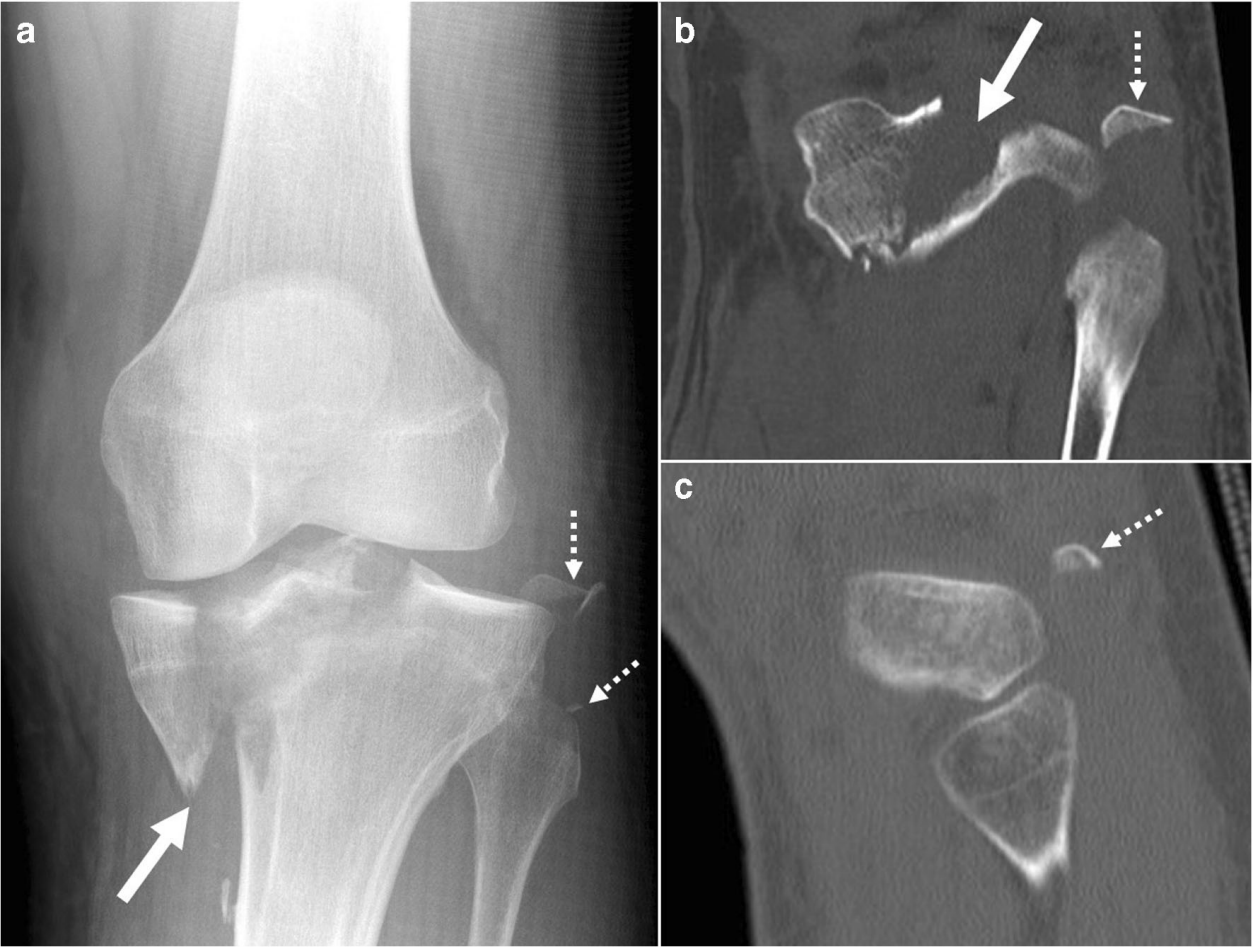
Radiograph (a), CT coronal (b), and sagittal (c) images of 34-year-old male patient who injured his leg by twisting it while playing beach volleyball, show a B3.2 medial plateau fracture (*arrow*) and a fibular head fracture (*dashed arrow*)
